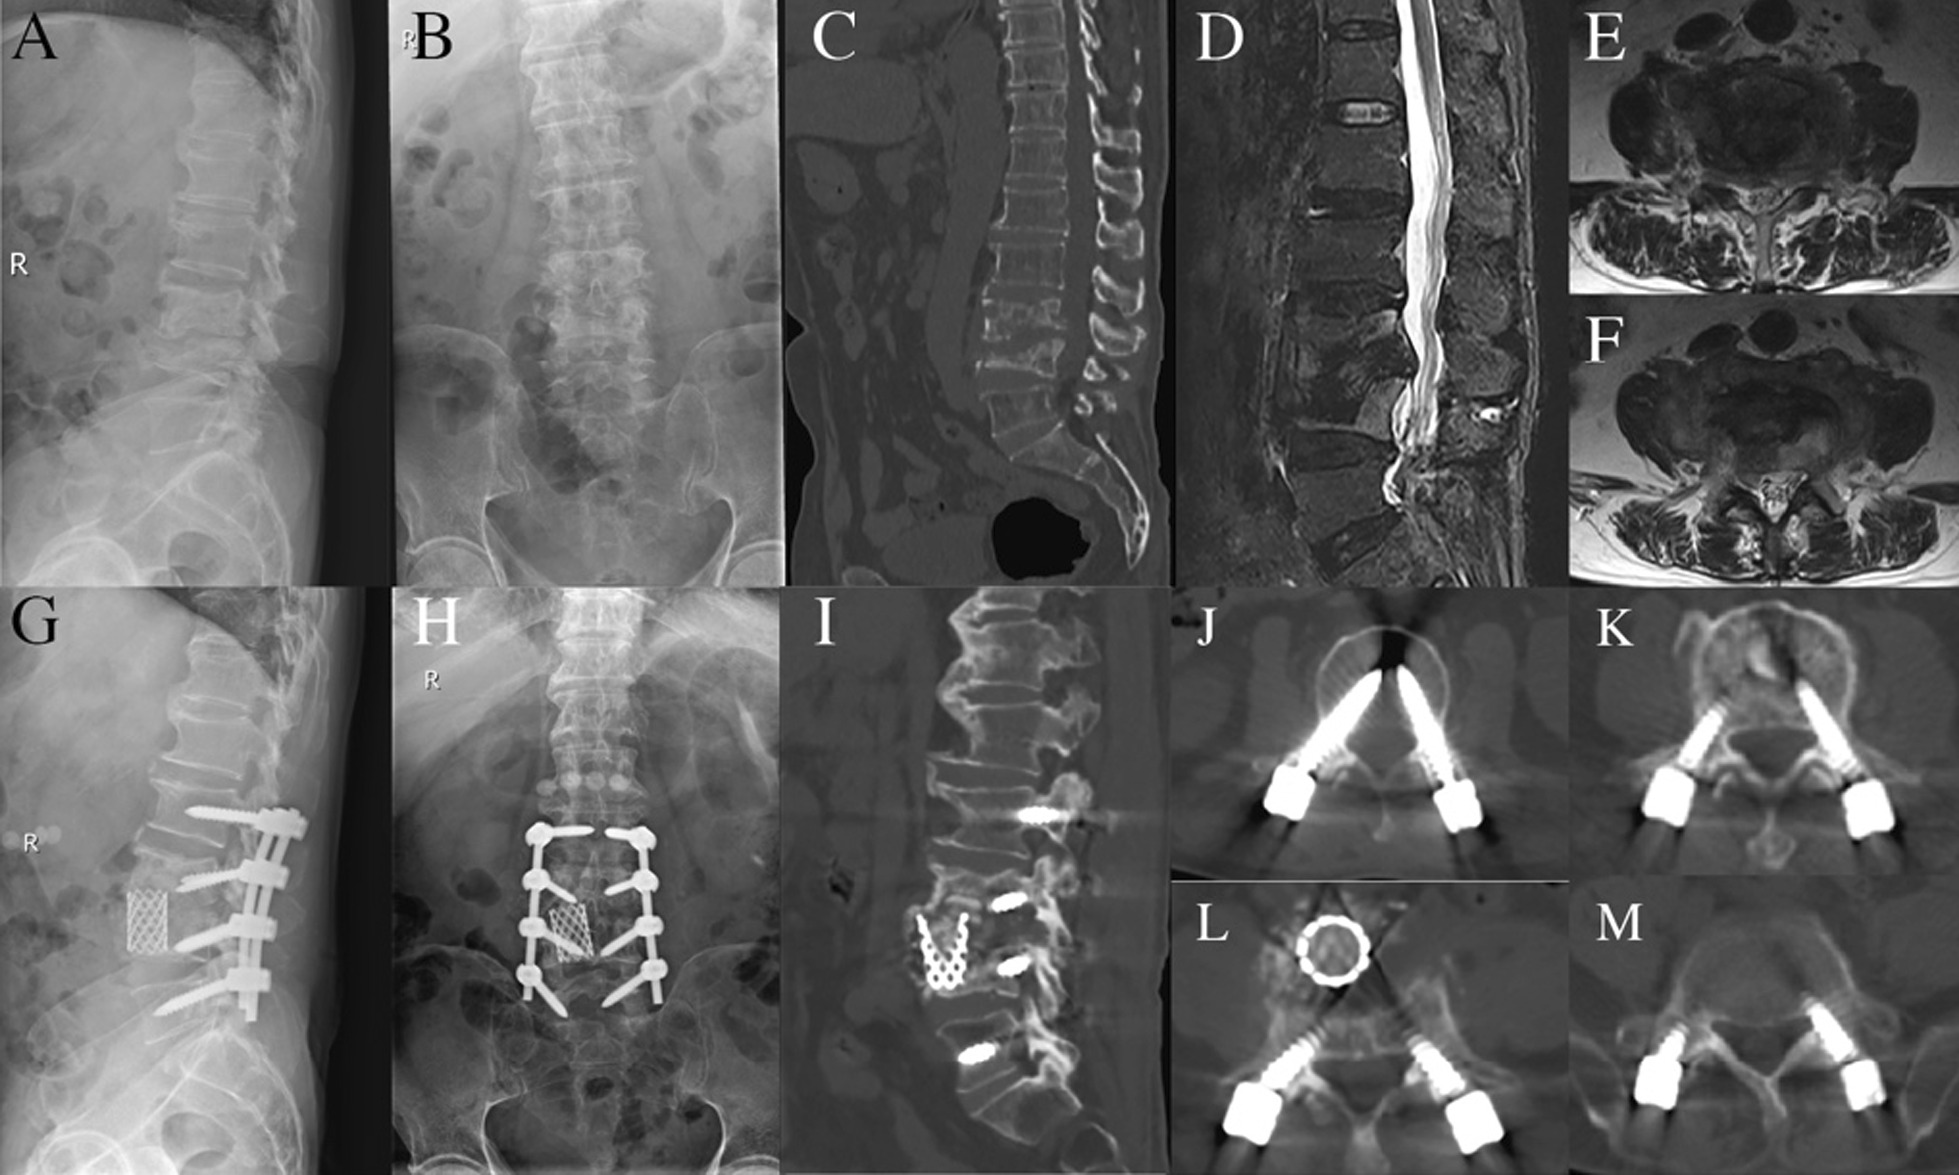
Radiographic images of a representative case diagnosed as L3, 4 tuberculosis. A, B preoperative X-ray in lateral and AP view. C preoperative CT in lateral view showing the obvious bony destruction in L3, 4; D, E, F MRI in lateral and cross-sectional view showing obvious abscess formation and severe bony defect; G, H postoperative X-ray in lateral and AP view showing reconstruction with a titanium mesh in L3/4 and multisegmented fixation from L2–5; I, J, K, L, M postoperative CT scan in lateral view and cross-sectional views showing the mesh and screws in place. *AP* Anterior–posterior
